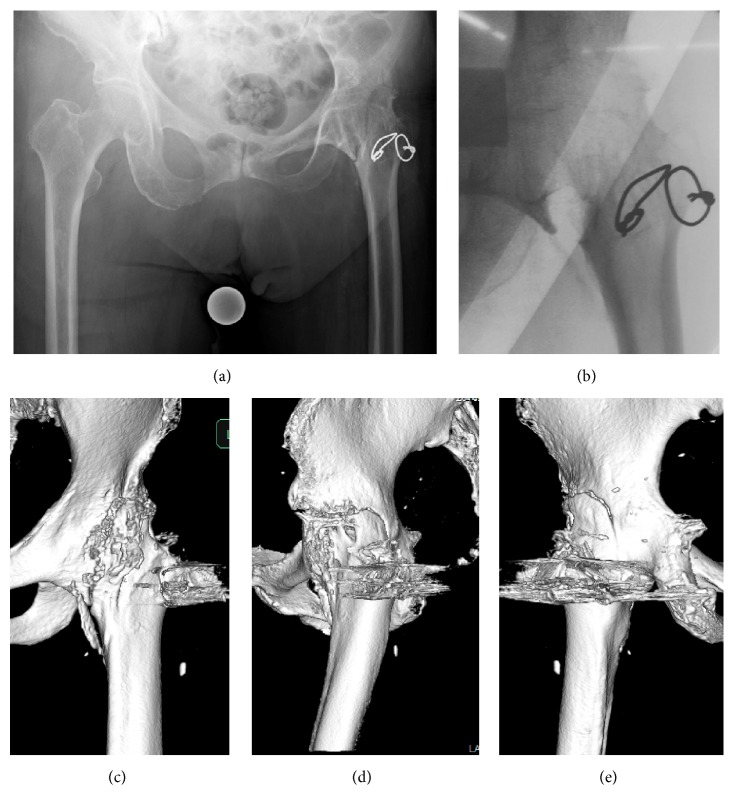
Preoperative images of the hip. (a) Radiograph of the anteroposterior (AP) view. (b–d) 3D-CT: (b) AP, (c) lateral, and (d) posterior-anterior (PA) views of the hip. Proximal femoral fracture in arthrodesed hip. Fracture line extends to pelvis. (e) Intraoperative image by intensifier. Instability of fracture site was confirmed and the displacement was increased with hip adduction.
